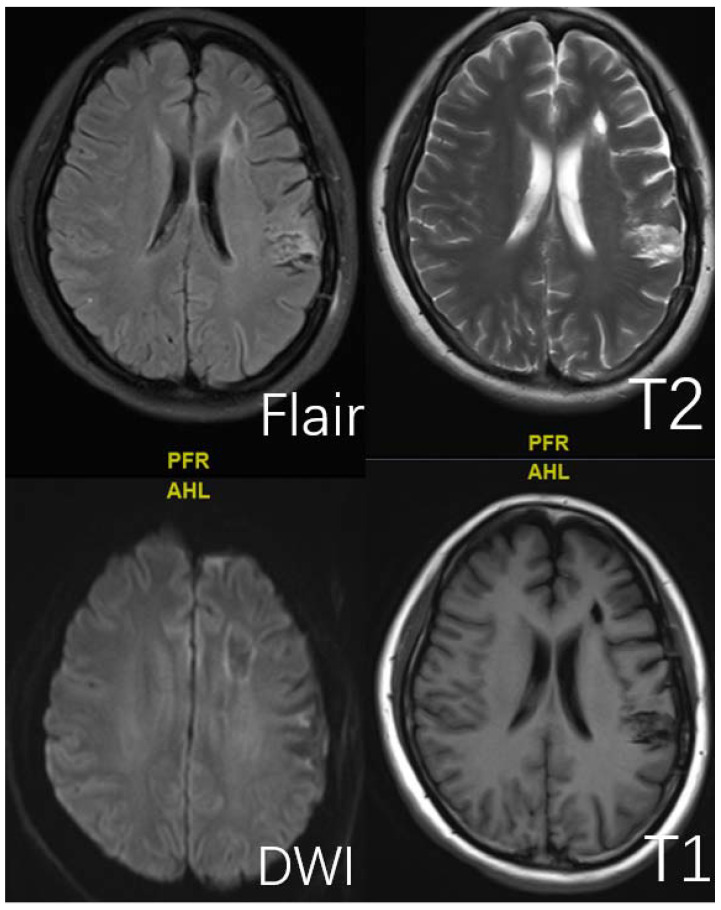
This is a 45-year-old female. Typical presentation of old cerebral infarction on T1, T2, DWI, and Flair sequences.
